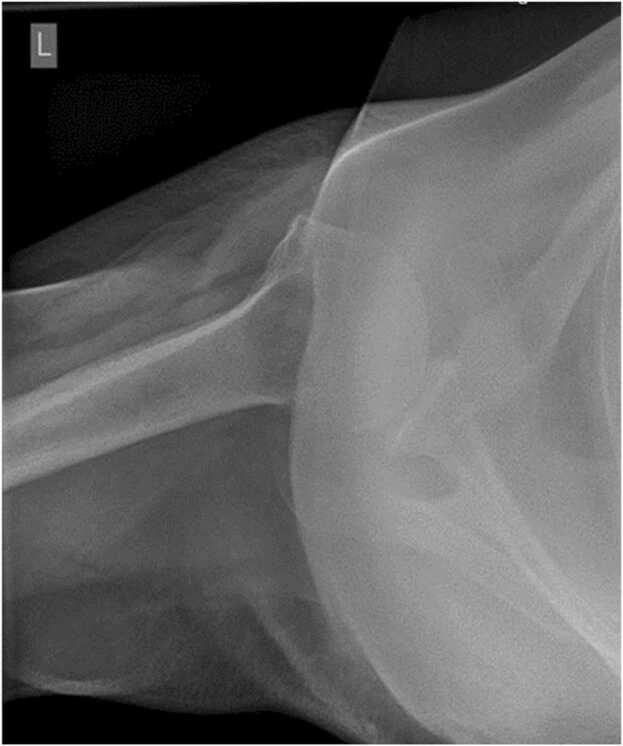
Plain radiograph of the shoulder (Axial view) showing joint space widening and acromioclavicular joint osteoarthritis.
